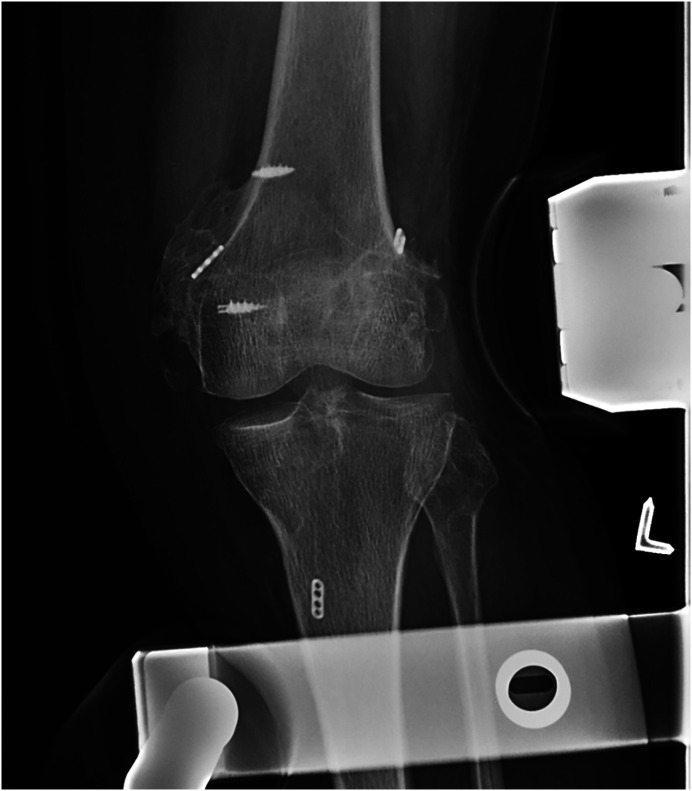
Radiographic valgus stress view of a patient with a KDIIIM, showing heterotopic ossification around the medial epicondyle at 9 months postoperatively.
